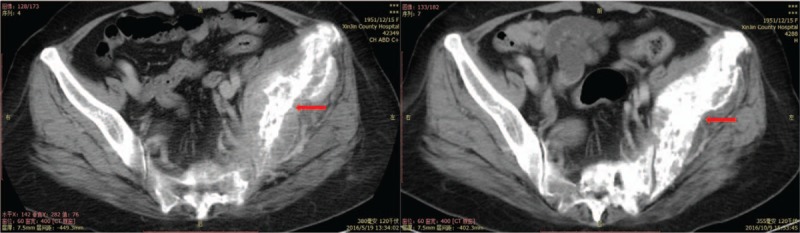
Computed tomography shows the mass in the left pelvis before and after treatment.
